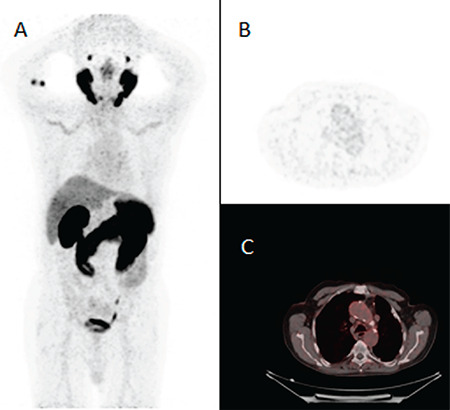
A-56-year-old male patient diagnosed with prostate cancer was referred to nuclear medicine for gallium-68 (^68^Ga) prostate-specific membrane antigen (PSMA) positron emission tomography/computed tomography (PET/CT) imaging. An intravenous solution of 4 mCi ^68^Ga PSMA was administered followed by whole-body PET/CT imaging at 1 hour post administration of intravenous solution. No recurrence or metastatic PSMA uptake was observed for prostate cancer. However, increased PSMA uptake was noted bilaterally in the subclavian arteries and common carotid arteries in maximum intensity projection (A), axial PET (B), and axial fusion (C) images. PSMA is a type 2 transmembrane protein with high expression in prostate carcinoma cells (1). ^68^Ga PSMA PET/CT has an important role in the assessment of patients with prostate cancer and recurrence and metastasis of the disease (2). ^68^Ga PSMA uptake has been evident in various solid malignant neoplasms such as neuroendocrine tumors, renal cell carcinoma, breast cancer, and differentiated thyroid cancer (3). This form of vessel uptake can be seen with ^18^fluorine-fluorodeoxyglucose (^18^F-FDG) PET in vasculitis. This patient was diagnosed with giant cell vasculitis. Recognition of the potential sources of false-positive and false-negative findings is important for accurate interpretation of PSMA-targeted PET imaging studies.
